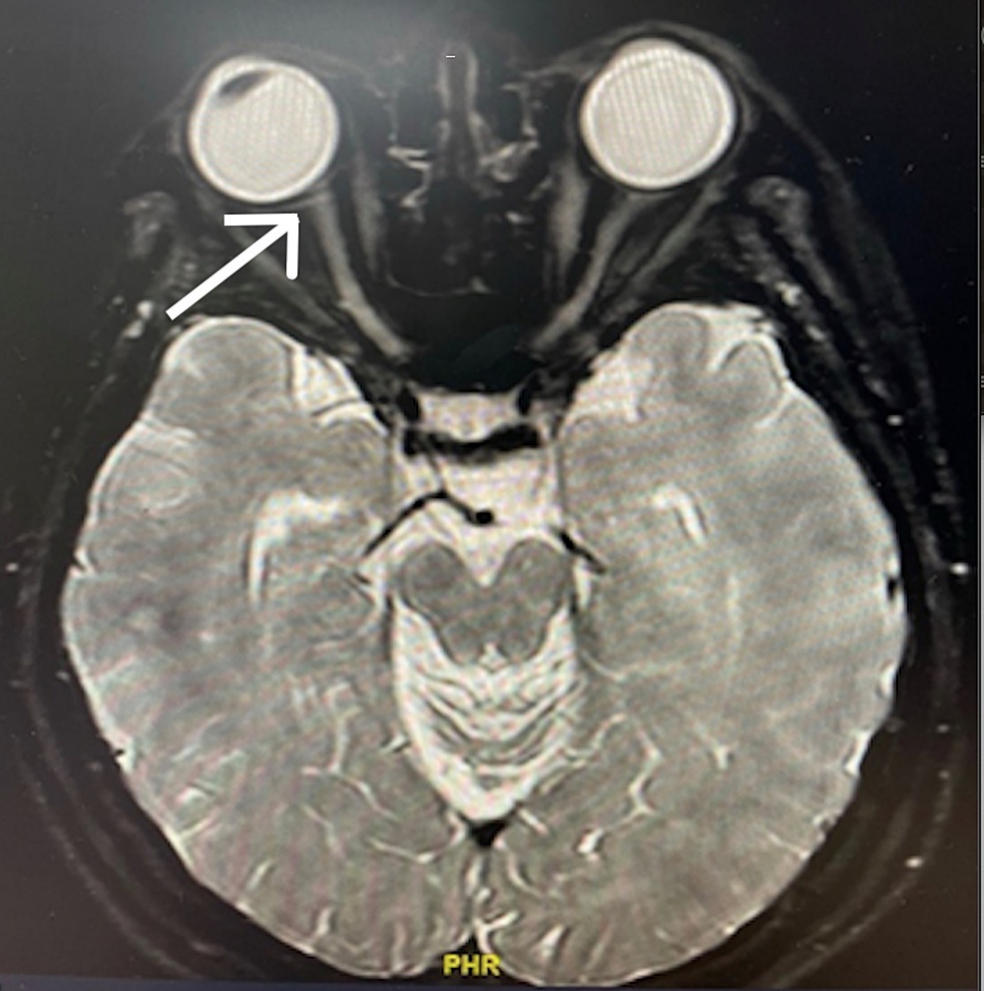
MRI of the brain and orbit White arrow: normal optic nerve, with no enhancement noted
